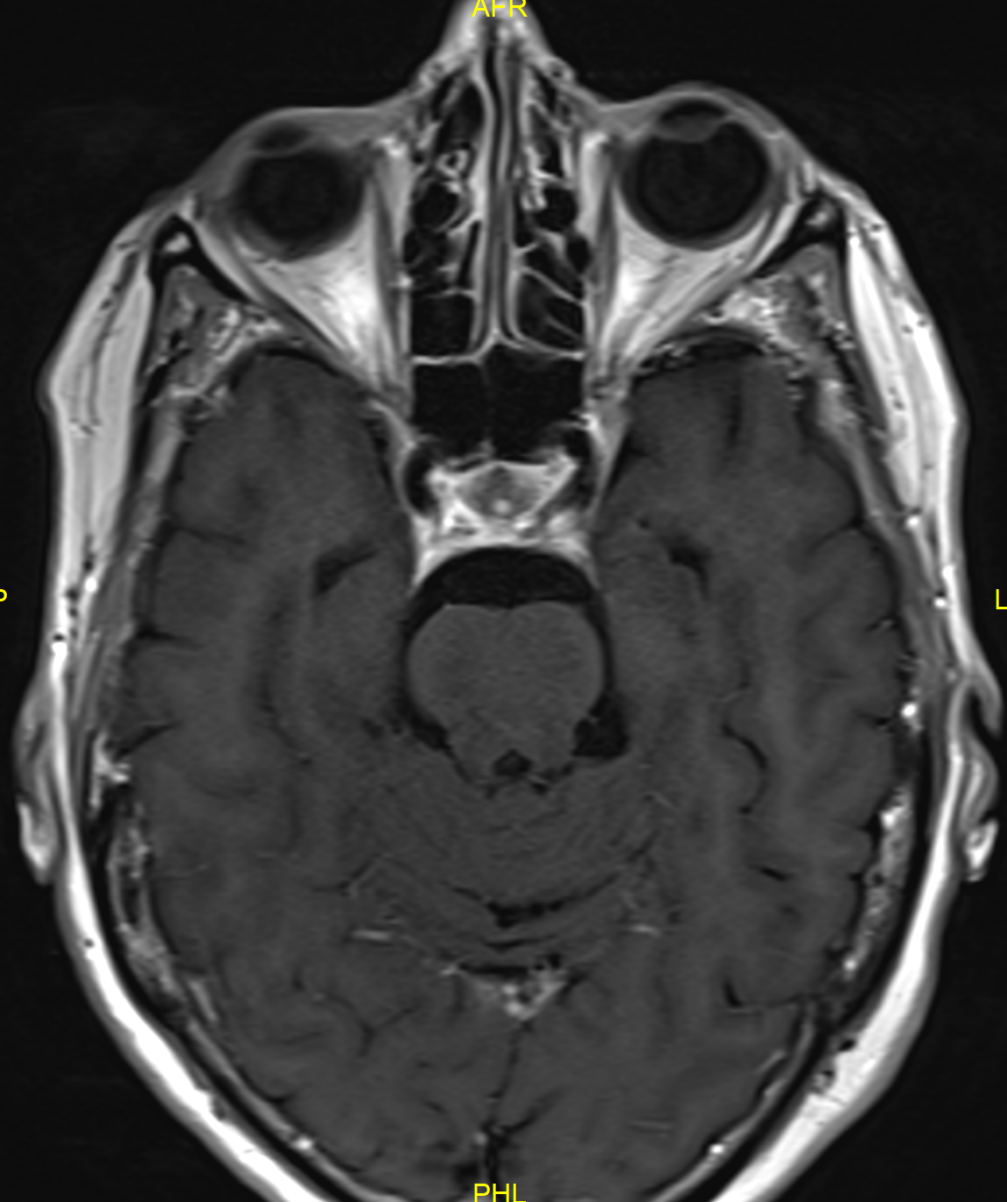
MRI (2020) at the level of the cavernous sinus
